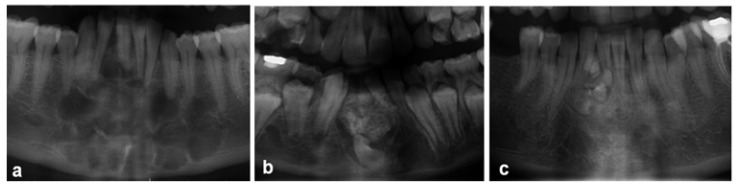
Examples of cropped panoramic radiographs of patients with odontogenic tumors: a) Odontogenic myxoma, b) Ameloblastik fibro-odontom; c) Odontoma.
